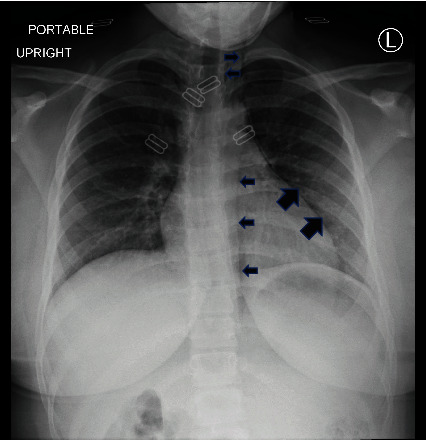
Chest X-ray upon admission to the emergency department demonstrates pneumomediastinum without pneumothorax (solid arrows).
